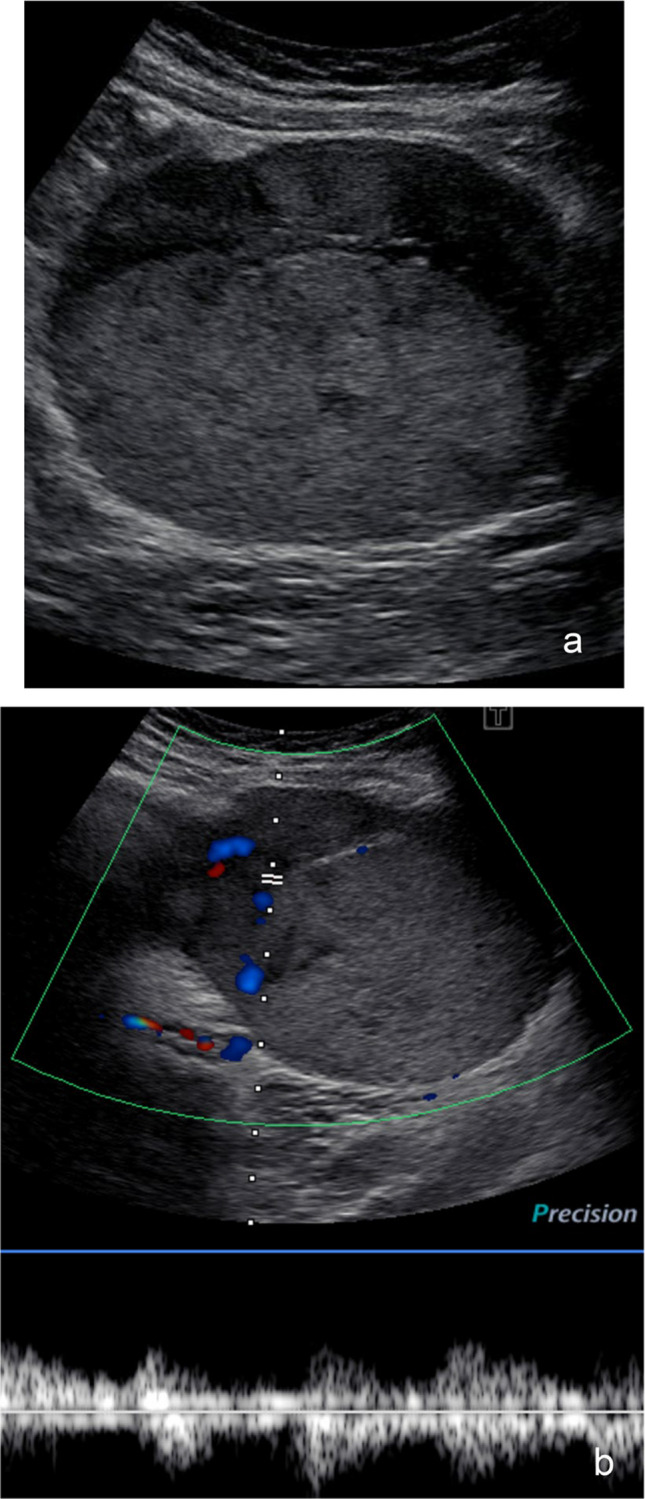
Ultrasound image example of a true positive ovarian/adnexal lesion which all 3 readers labeled correctly in a 10-year-old female: Longitudinal static grayscale (a) and color Doppler (b) ultrasound images demonstrates a 9.5-cm solid appearing lesion of the right adnexa with smooth outer contour and heterogeneous hyperechoic internal echotexture. Color and spectral Doppler demonstrate vascular flow with low resistance arterial waveforms (color score, CS=2). No shadowing was identified. The three reviewers labeled this lesion as O-RADS 4 (×2) and O-RADS 5 (×1) and was pathologically proven to represent a dysgerminoma
